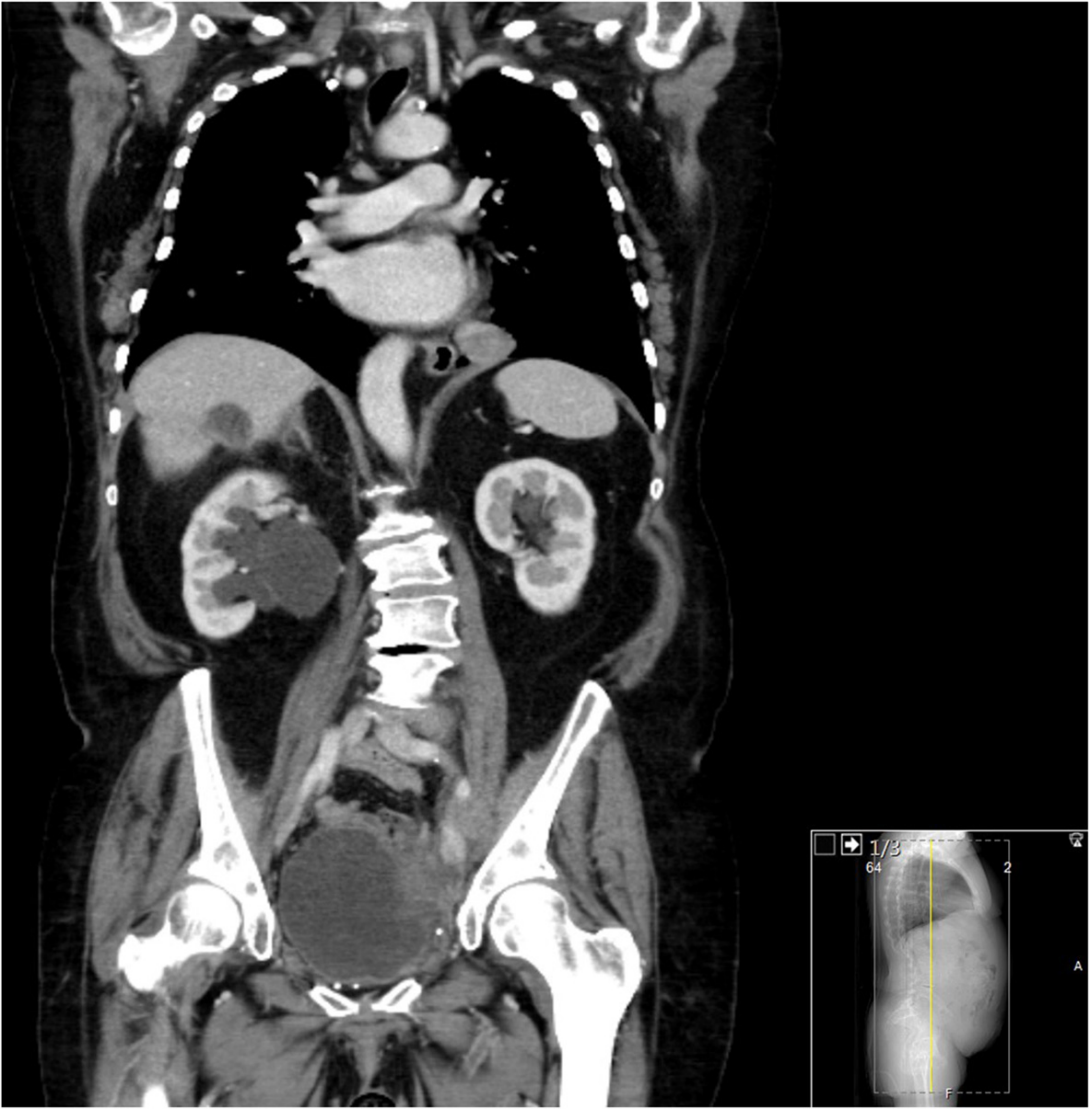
Preoperative CT before the second operation, showing the hepatic mass in segment 7 in the coronar plane
